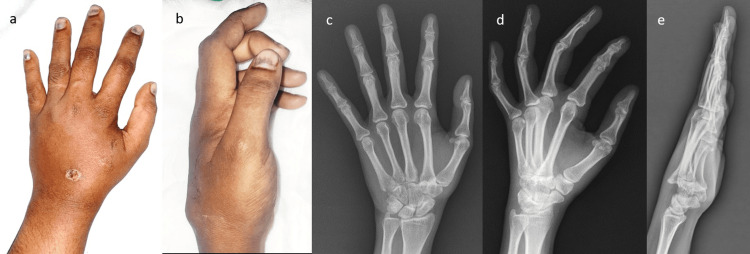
Clinical pictures and X-rays at presentation (a) and (b) clinical pictures showing swelling over the dorsum of the hand, (c) anteroposterior and (d) oblique view of hand showing minimal bony overlap at second carpometacarpal joint, (e) lateral view showing dorsal dislocation at second carpometacarpal joint
